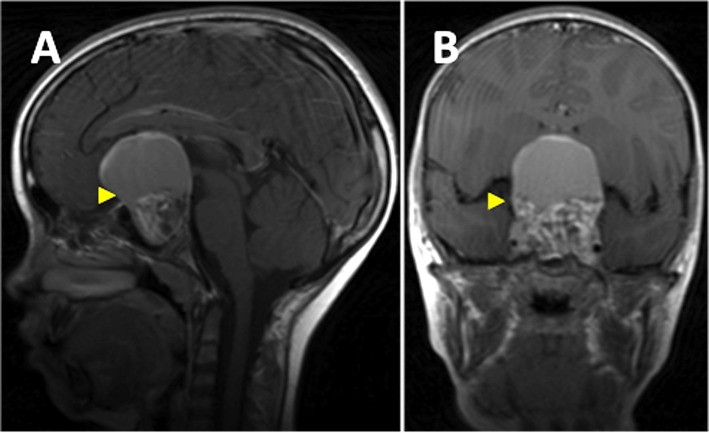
T1-weighted MRI brain with contrast (sagittal view [panel A], coronal view [panel B]) Shows a large partly cystic, partly solid craniopharyngioma (arrowhead) with suprasellar extension elevating the optic chiasm, third ventricle, and causing sellar expansion.
